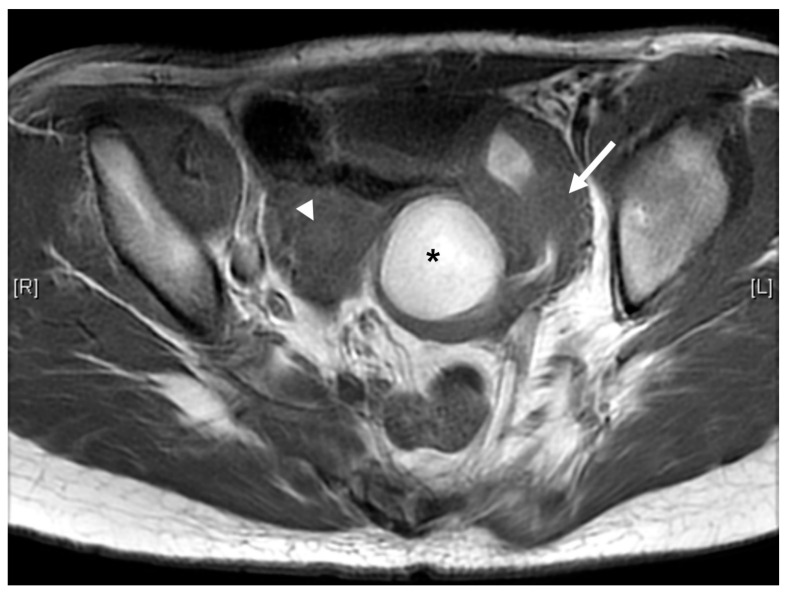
MRI of case 3: hematometrocolpos (asterisk) and uterine didelphy (arrow and arrowhead).
